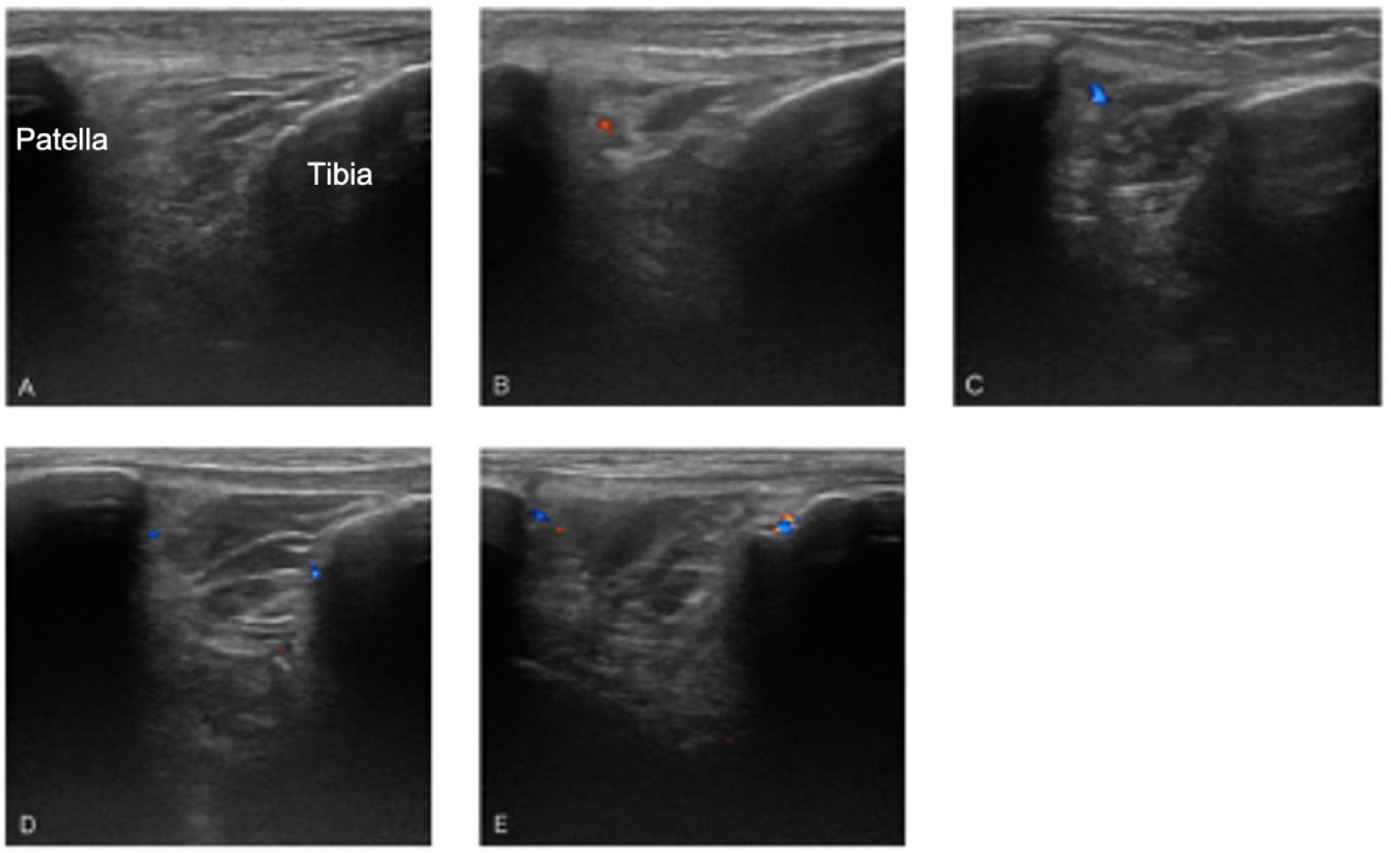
Representative Color Doppler signals. (A) shows no blood signals were observed in the IPFP (grade 0). (B) and (C) Single vessel signal was observed in the IPFP (grade 1). (D) and (E) More than two vessel signals in less than half of the area of the IPFP (grade 2). Prior to acquisition of data, two examiners examined five randomly selected subjects to assess inter-reproducibility of ultrasound examination. Perfect agreement was observed.
